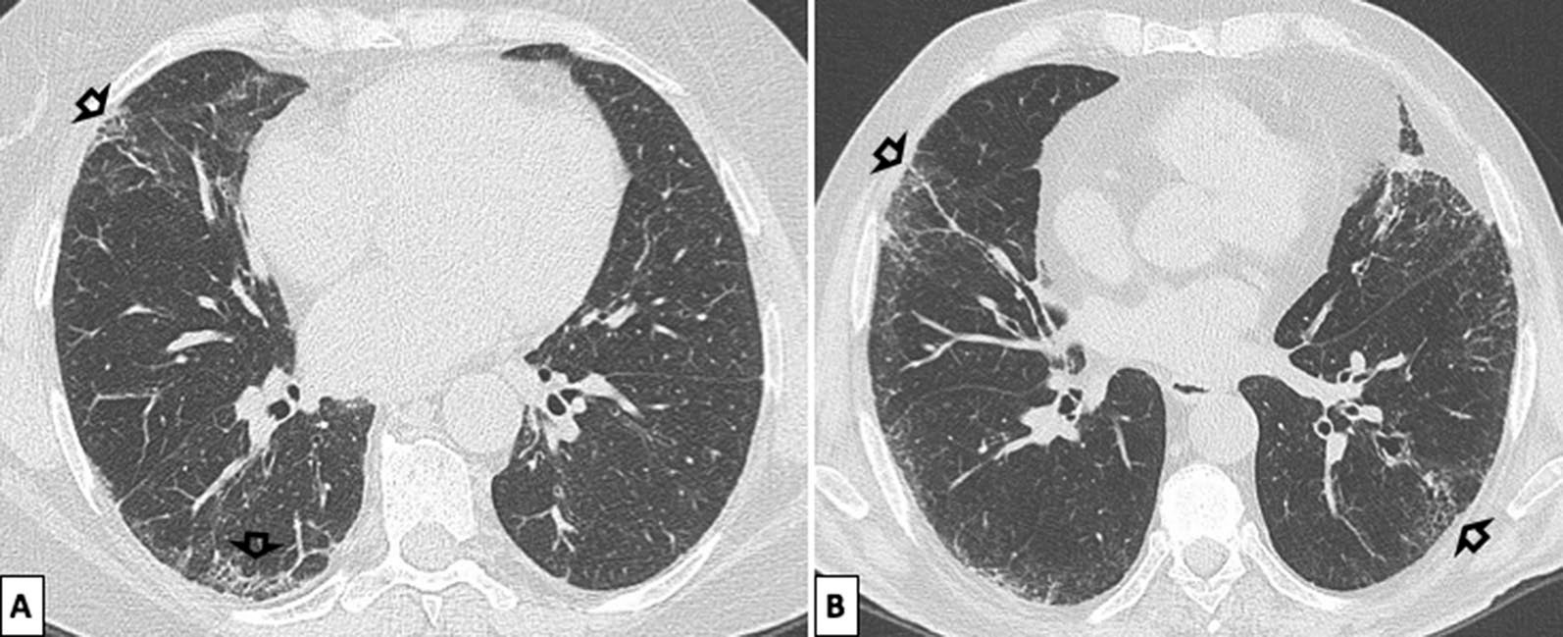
a, b Axial CT images without contrast (slice thickness of 1 mm) of a 77-year-old gentleman show bilateral GGOs and reticular opacities (*open arrows*) involving the subpleural regions of both upper and lower lobes. This pattern is compatible with non-fibrotic subpleural ILAs, since no overt signs of fibrosis, such as bronchiectasis or honeycombing, are displayed
